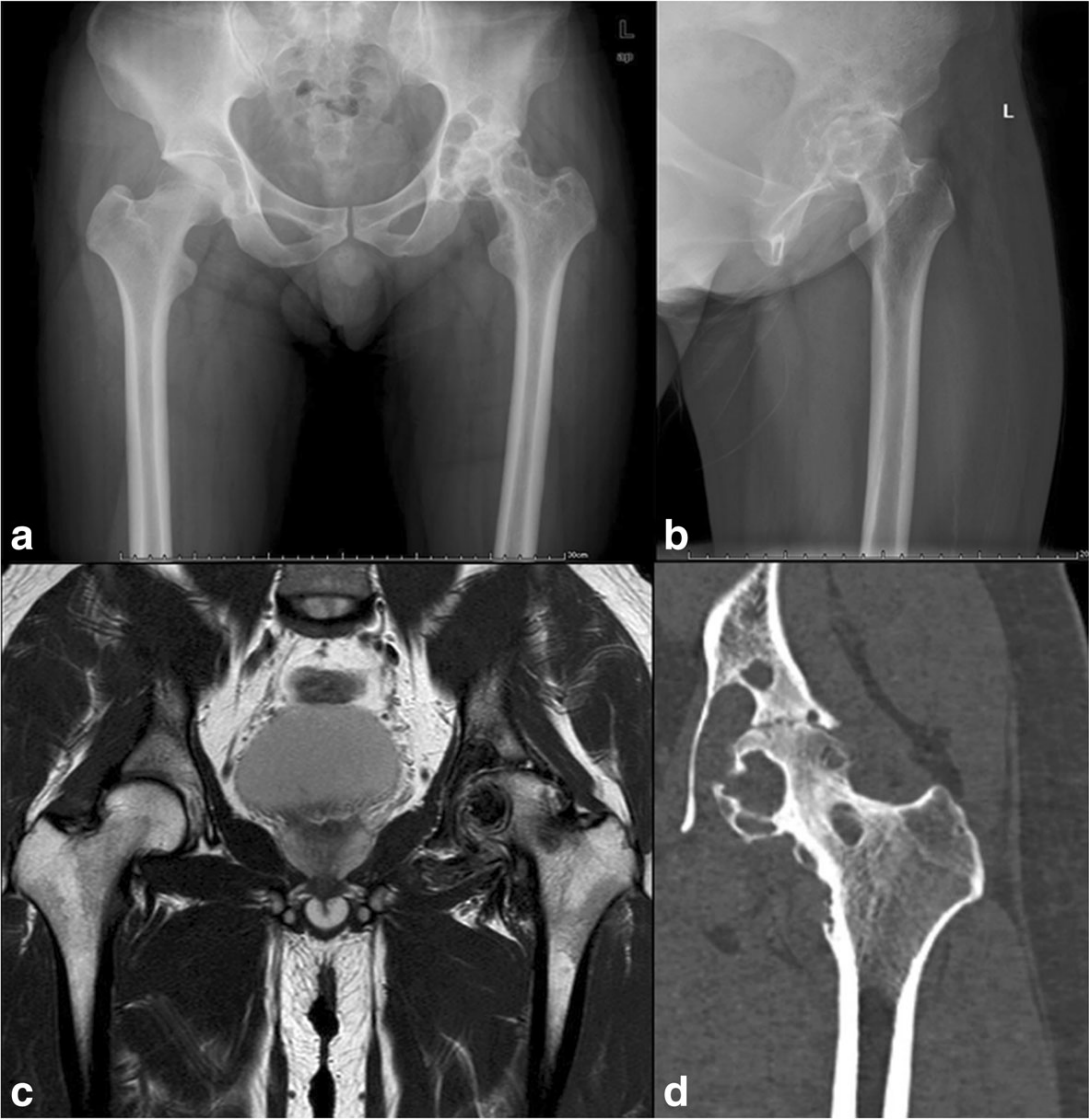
The typical appearance of PVNS as seen in radiography of the hip, magnetic resonance imaging (MRI), and computed tomography (CT). Anteroposterior radiograph of case 5 (a) and lateral radiograph of the case 16 (b) show erosions and cysts in both the femoral head and the acetabulum of left hip with nearly complete obliteration of the joint space. CT (c) and MRI (d) of case 14 show the characteristic cyst-like structure of the femoral head
